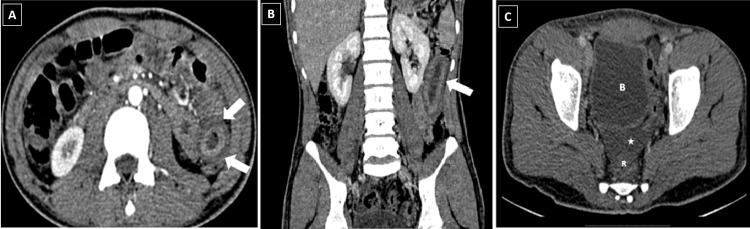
Contrast-enhanced CT scan Figure A (axial) and Figure B (coronal reformats) are contrast-enhanced CT scan images showing the presence of bowel wall thickening with submucosal edema producing the target appearance (arrows). Note the intense enhancement of the mucosal surface. This abnormality was segmental, involving one of the proximal jejunal loops. Figure C shows free fluid in the pelvis (star). B: bladder; R: rectum
